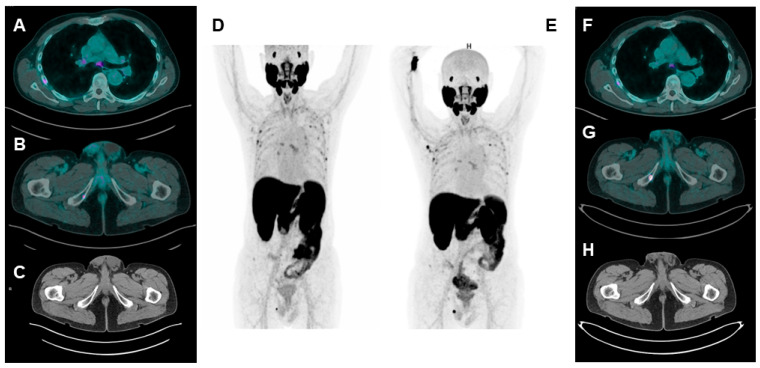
A patient with biochemical recurrence of PCa undergoing [^18^F]F-PSMA-1007 PET/CT (PSA = 0.22 ng/mL). Axial fused (A,B), CT axial (C), and maximum intensity projection (MIP) (D) images show multiple spots of focal uptake involving several ribs and the right ischio-pubic branch. The low PSA value leads to suspicion of UBU; therefore, a second [^18^F]F-PSMA-1007 PET/CT is performed 6 months later ((E): MIP image; (F,G): axial fused images; (H): CT axial image). While the rib uptakes appear stable for number, size and uptake intensity are suggestive of UBU; the uptake at the right ischio-pubic branch is considerably increased and an osteo-structural alteration can be detected at CT images. Combining imaging suspicion with increased PSA value (1.20 ng/mL), the lesion at the right ischio-pubic branch is considered a PCa metastasis.
